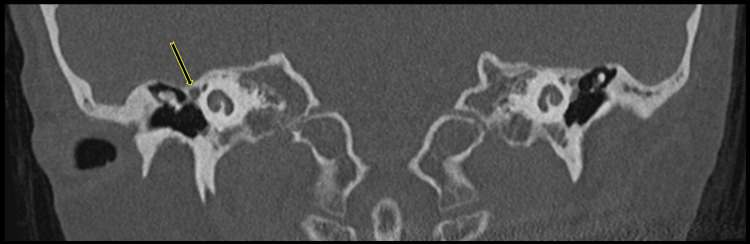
Temporal bone coronal CT reconstructions demonstrate asymmetrical enlargement of the tympanic segment of the right facial nerve canal. Asymmetrical enlargement of the tympanic segment of the right facial nerve canal (long arrow), harboring both PSA and the tympanic segment of the facial nerve. PSA: persistent stapedial artery
